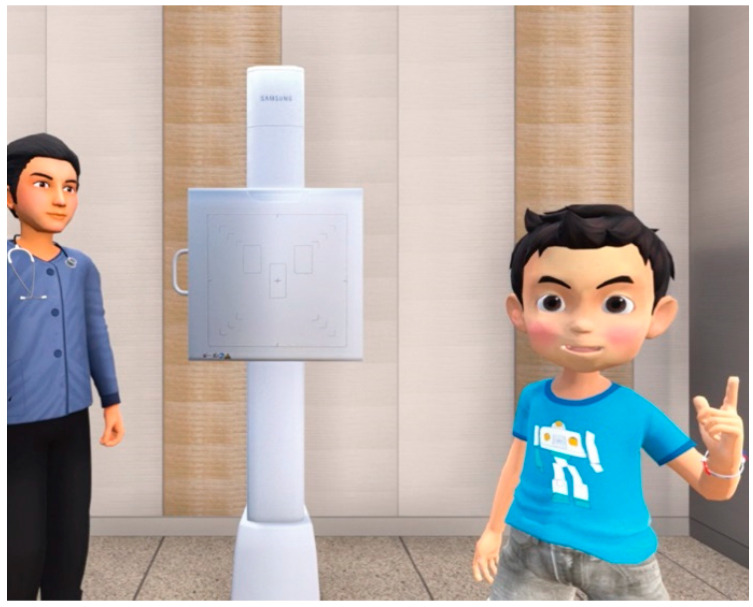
Chatan explaining how to cooperate appropriately for chest radiography.
